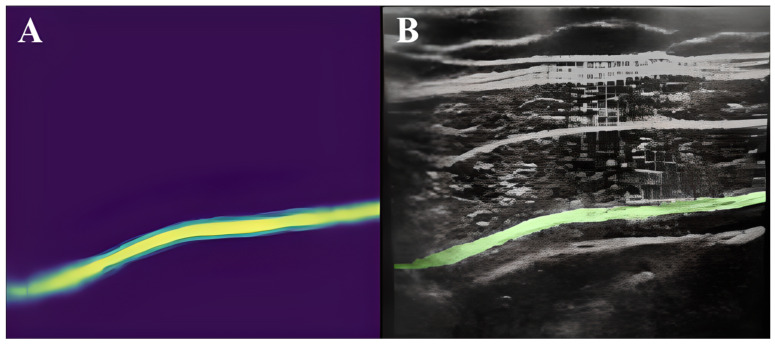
Transverse abdominis (green color) model prediction on a randomly selected image (A) and labeling on the ultrasound image (B) (from [11]).
